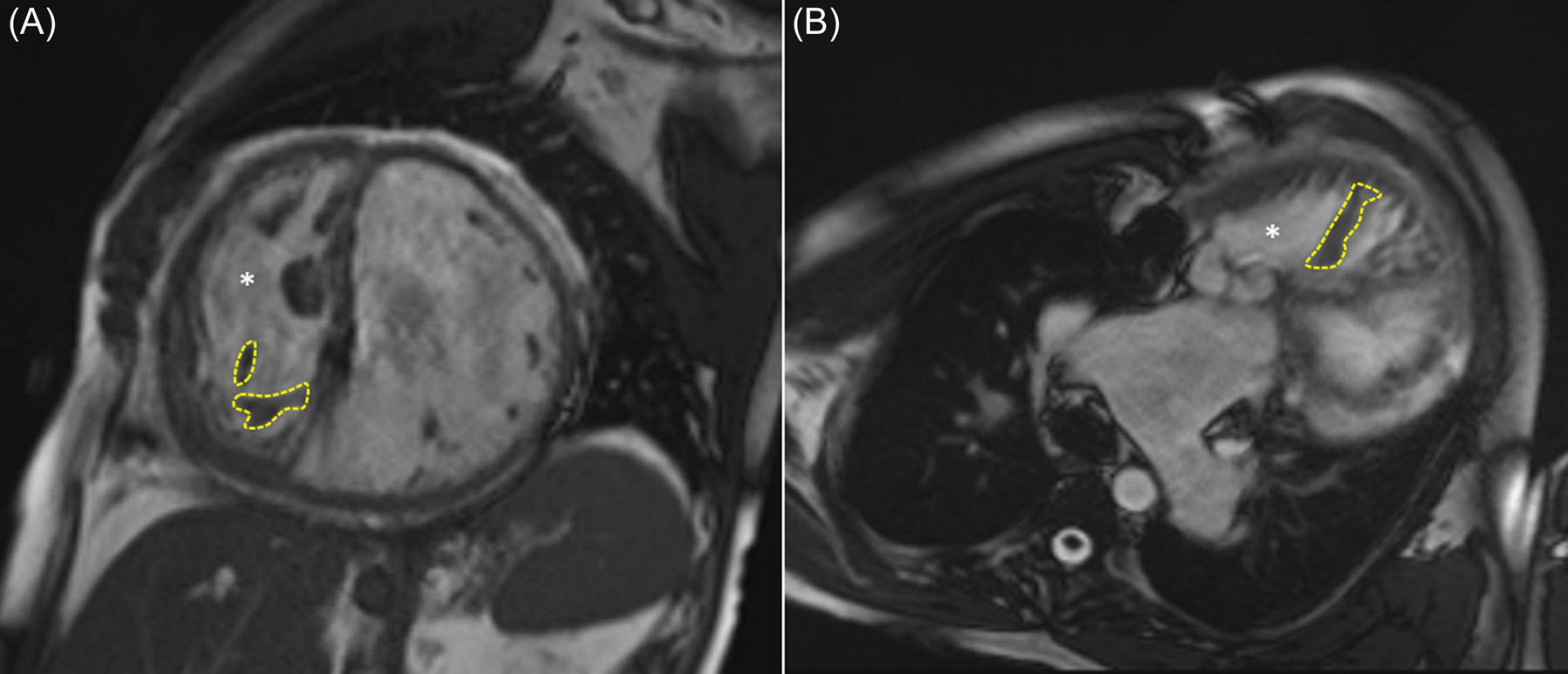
Cardiac MRI characterizing right ventricular morphology. (A) Short‐axis view seen from a sagittal cut shows a dilated RV and LV. *indicates the RV. The structure outlined in dotted yellow are the papillary muscles along the diaphragmatic free wall of the RV. (B) A representative slice of the four‐chamber view from a transverse cut. MRI, magnetic resonance imaging; LV, left ventricle; RV, right ventricle.
